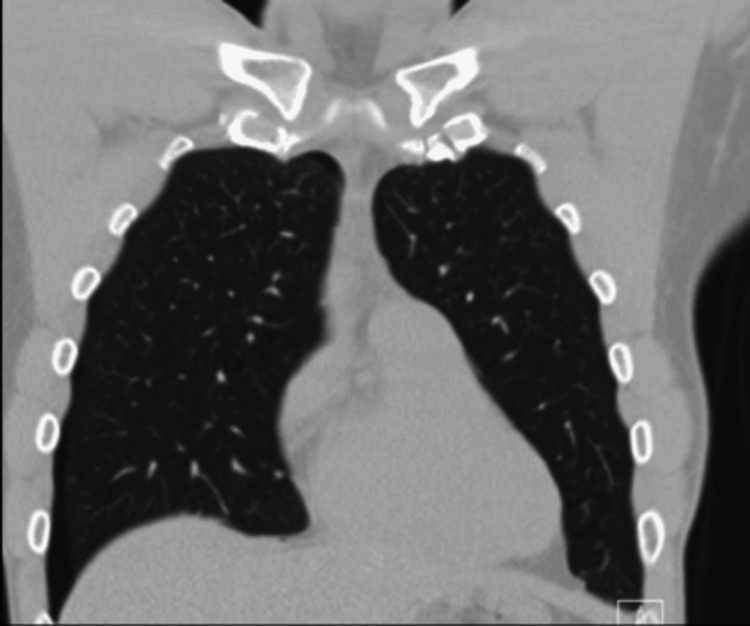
CT scan showing right apical and anterior pneumathoraxes (coronal view)
